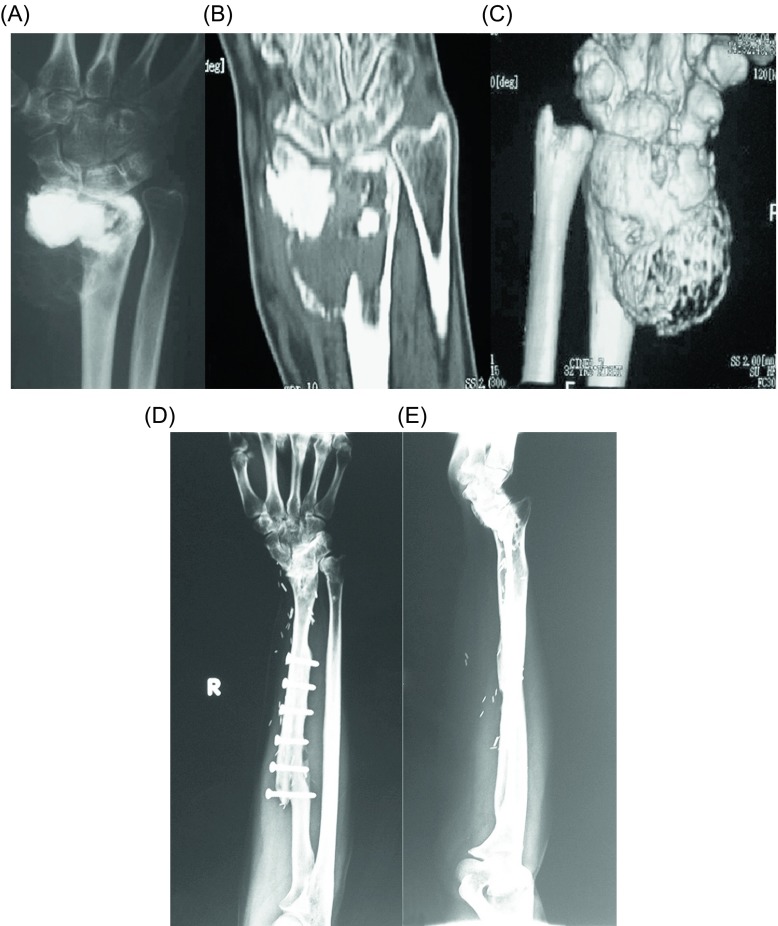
(A) Anteroposterior radiograph, (B) coronal CT, and (C) three-dimensional CT reconstruction of the right wrist of a 40-year-old man with a recurrent GCT of the distal radius after curettage and PMMA cementation. Wide resection and free vascularized fibula graft distal radius reconstruction were done. (D) Anteroposterior and (E) lateral radiographs of the right wrist show no evidence of local tumor recurrence at 16-year follow-up.
